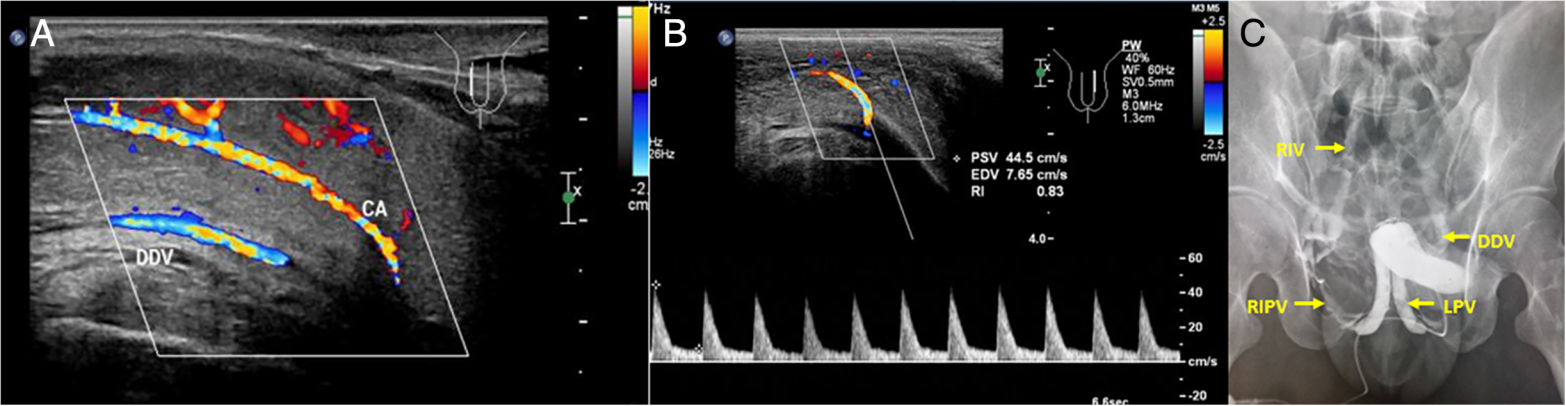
Mixed venous leak in a 26‐year‐old man. A, The continuous blood flow signal was observed in the deep dorsal vein (DDV) after intracavernosal injection by ultrasonography. B, The cavernous arterial (CA) spectrum showed PSV > 30 cm/s, EDV > 5 cm/s, and RI = 0.83. C, The contrast agent can be seen in the deep dorsal vein, the bilateral peduncular veins, the bilateral internal pudendal veins, the bilateral iliac veins, and even the right external iliac vein in the cavernographic image. DDV indicates deep dorsal vein; EDV, end‐diastolic velocity; LPV, left peduncular vein; PSV, peak systolic velocity; RIPV, right internal pudendal vein; RIV, right iliac vein.
